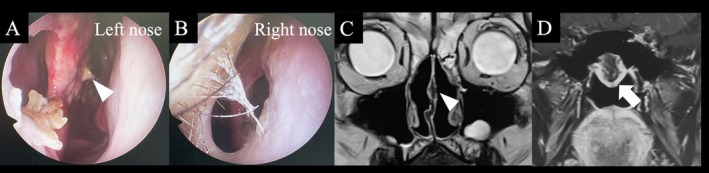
Endoscopic endonasal images after induction chemotherapy. Left (A) and right (B). Significant tumor reduction is observed. Residual tumor is noted in the posterior nasal septum (arrowhead). (C) (D) Coronal T2‐weighted magnetic resonance image obtained after induction chemotherapy. Significant tumor shrinkage is observed. A partially thickened area posterior to the nasal septum is suspected to have residual tumor (arrowhead), and there is clear residual tumor at the posterior end of the nasal septum (arrow).
